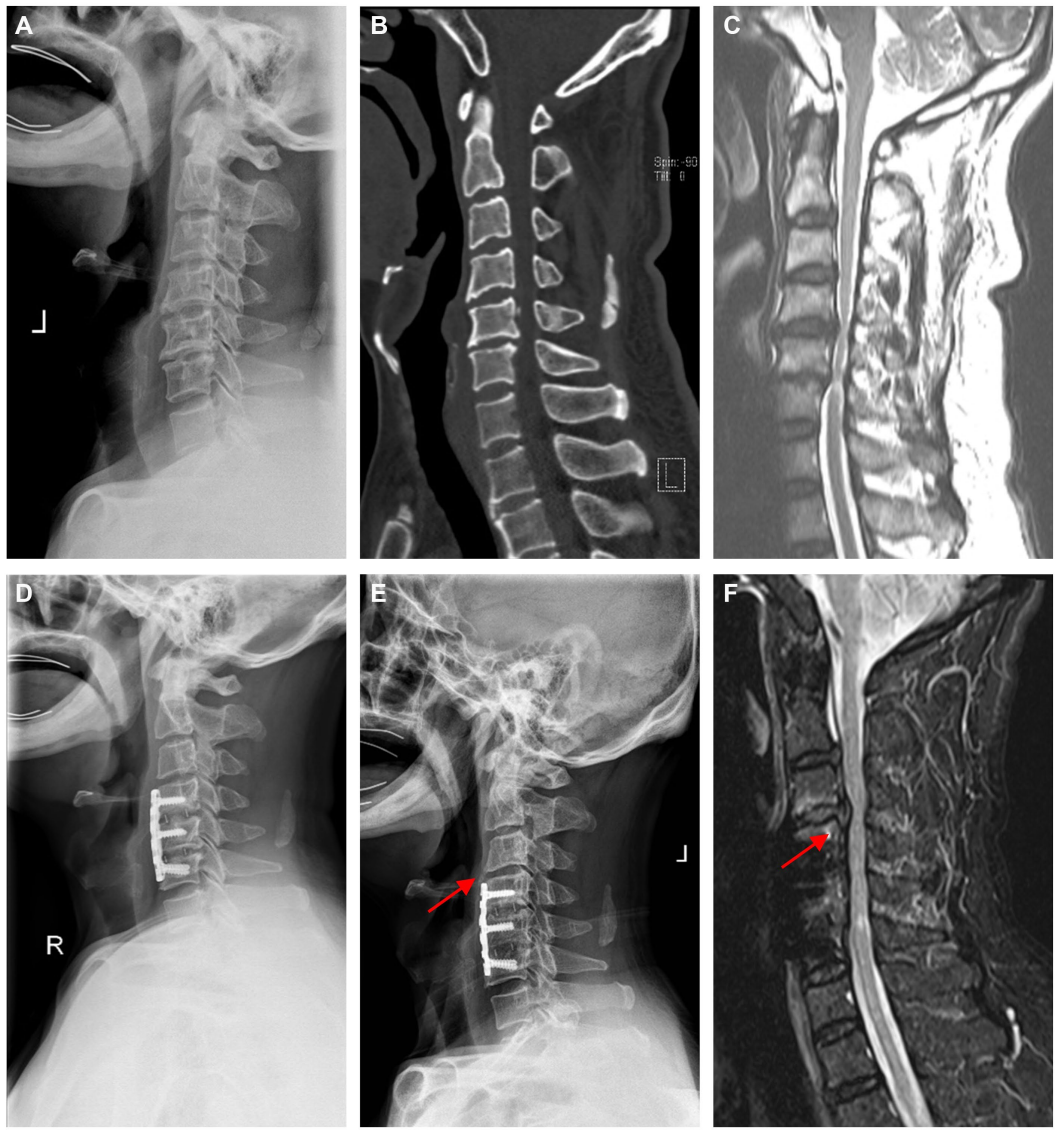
Images of a 66-year-old male patient who 79 months after anterior cervical plate and cage (ACPC) surgery. (A–C) Preoperative imaging revealed spinal cord compression at C4-C6, which was consistent with the symptoms. (D) Postoperative X-ray of 3 months indicates a relatively normal sequence of vertebrae. (E) At the last follow-up, the adjacent segment had decreased disc height and osteophyte formation anterior to the intervertebral space. (F) MRI suggested disc herniation over the operated segment, and clearly, compression of the spinal cord was observed at the last follow-up, as marked by the red arrow.
